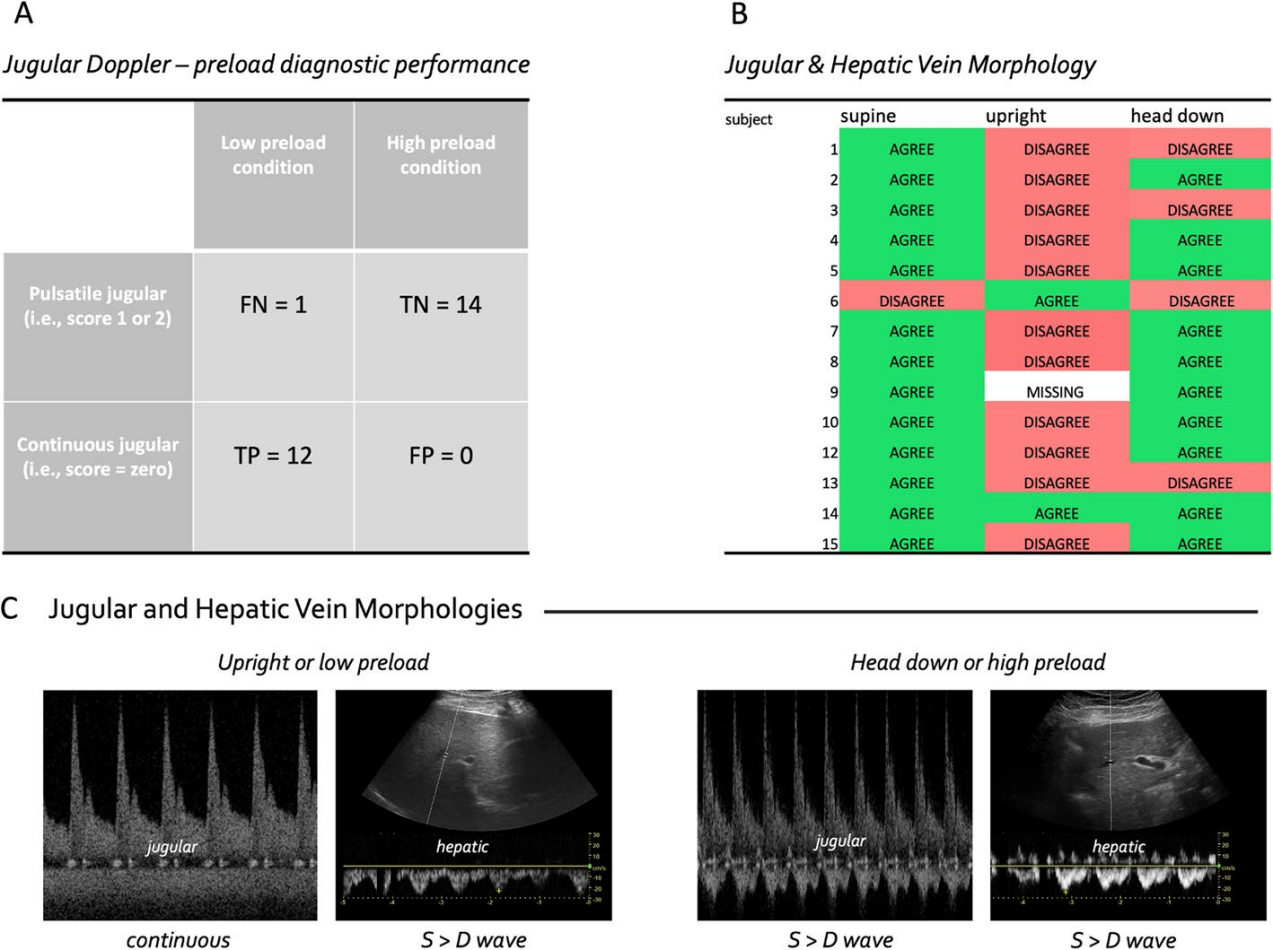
Effect of gravitational preload change on jugular and hepatic veins. A 2 × 2 diagnostic accuracy matrix for jugular morphology versus preload, FN is false negative, TN is true negative, TP is true positive and FP is false positive B agreement between the jugular vein and hepatic vein morphologies at different preload conditions. C Examples of jugular and hepatic vein Doppler at low (left most) versus high (right most) preload conditions
